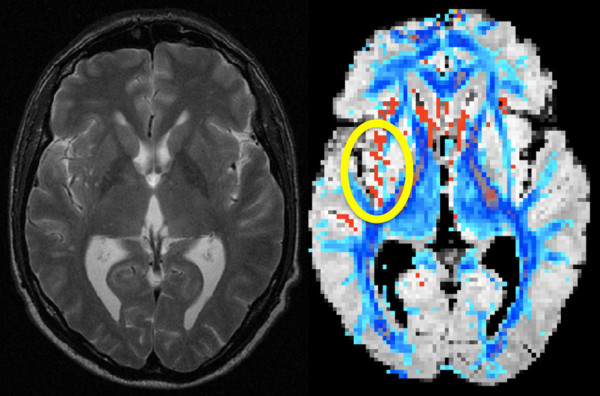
Example of typical macrostructural WM alterations on conventional structural MRI (A) and the correspondent FA changes (B). A: T2w image of one patient showing mainly unilateral hyperintense macrostructural lesions. B: Computer reconstructed hyperintensities of the same T2w dataset (red) overlaid with the patients FA (blue). Only FA values > 0.2 are shown. Close to the lesion (yellow circle) the FA is reduced (FA < 0.2) in comparison to the contralateral side. Similarly, uni- or bilaterally highly significant FA reductions were found in patients with macrostrucural lesions, as well as in patients without macrostructural WM hyperintensities.
